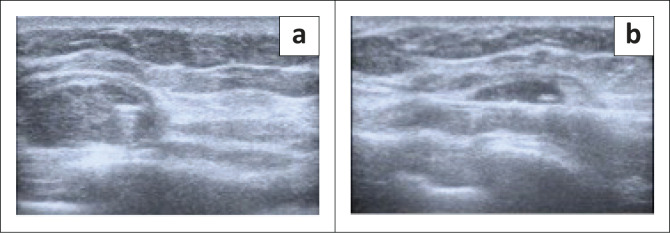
Post-surgical, axial (a) and sagittal (b) views of the left breast on ultrasound demonstrating the largest hypoechoic lesions at the inferior-lateral aspect of the scar. This lesion was not separable from the underlying pectoralis muscle.
